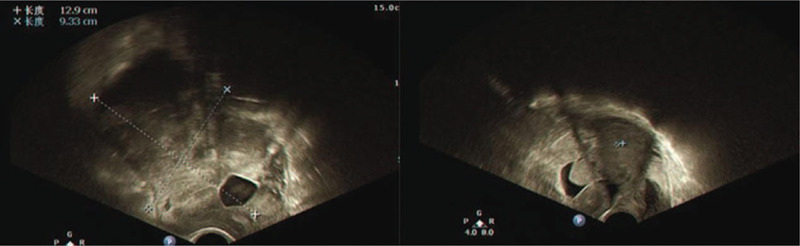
Ultrasound showed that in the right ovarian there was a mass consist of cystic and solid tissue with irregular shape, blurred boundaries and abundant blood flow signal inside.
